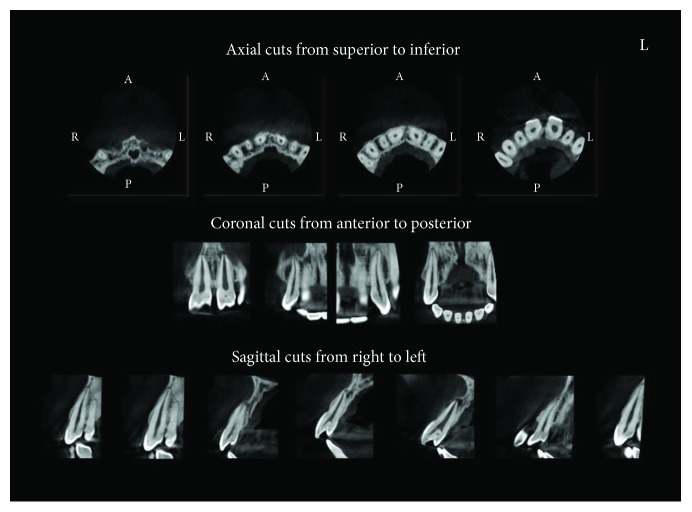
Cone beam computed tomography (CBCT) for the anterior segment of case 1.
